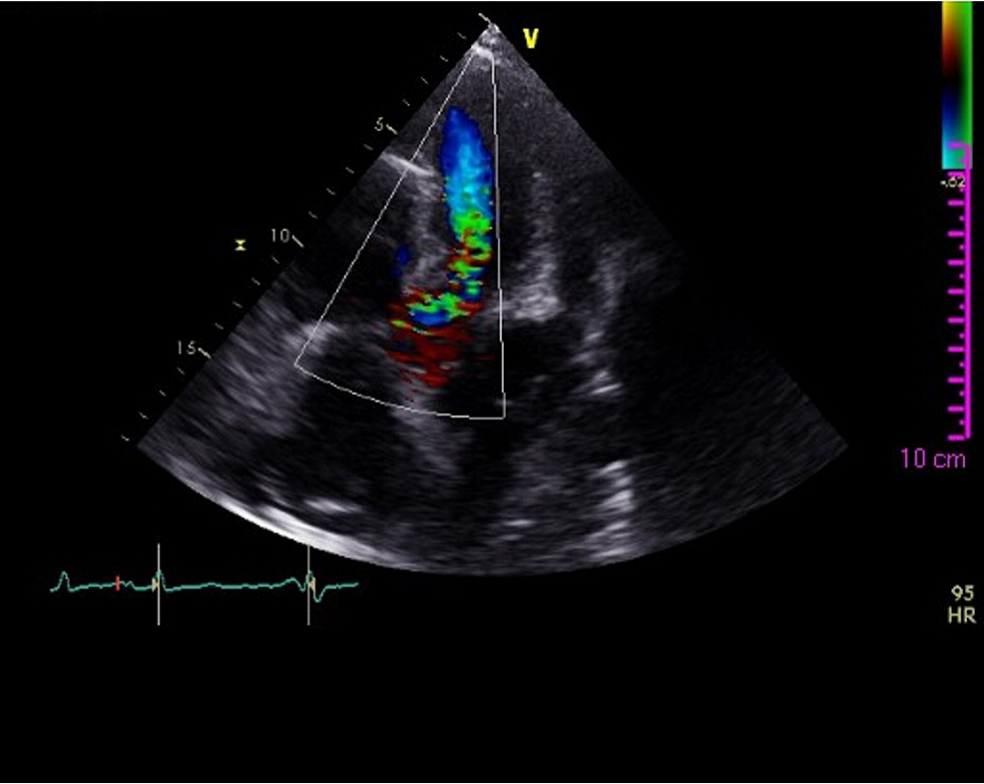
Transthoracic echocardiogram color-Doppler image of moderate to severe aortic regurgitation
